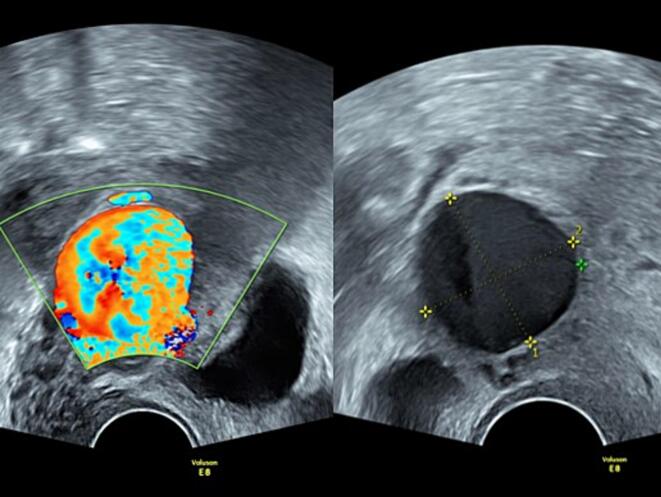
Initial presentation 5 weeks post-partum. Left: strong aliasing on Doppler in a round structure. Right: 27x27x30mm diameter round hypoechoic structure on B-mode.
